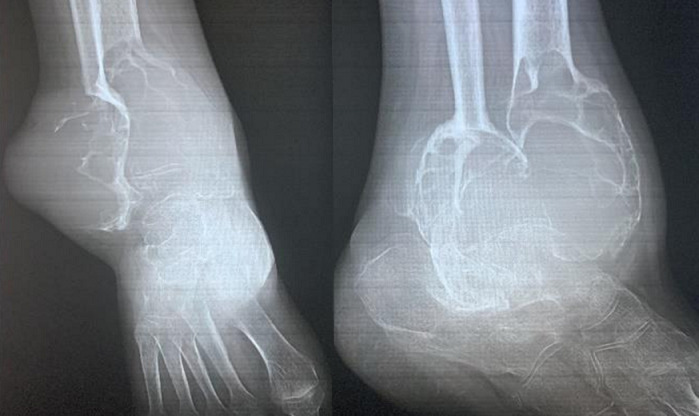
radiographie de la cheville gauche révélant une lésion ostéolytique extensive du quart distal du tibia et de la malléole externe, avec effraction corticale et extension dans les parties molles adjacentes
